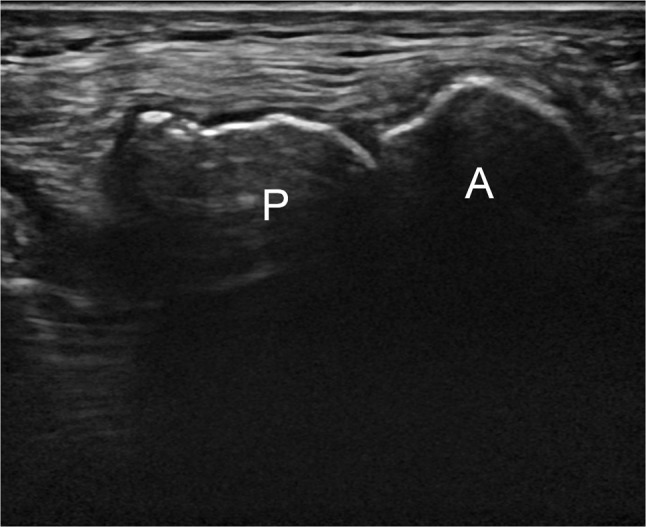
Ultrasound image of the patella, transverse viewpoint. Two patellar segments are visible: an anterior (A) and a posterior (P) segment.
